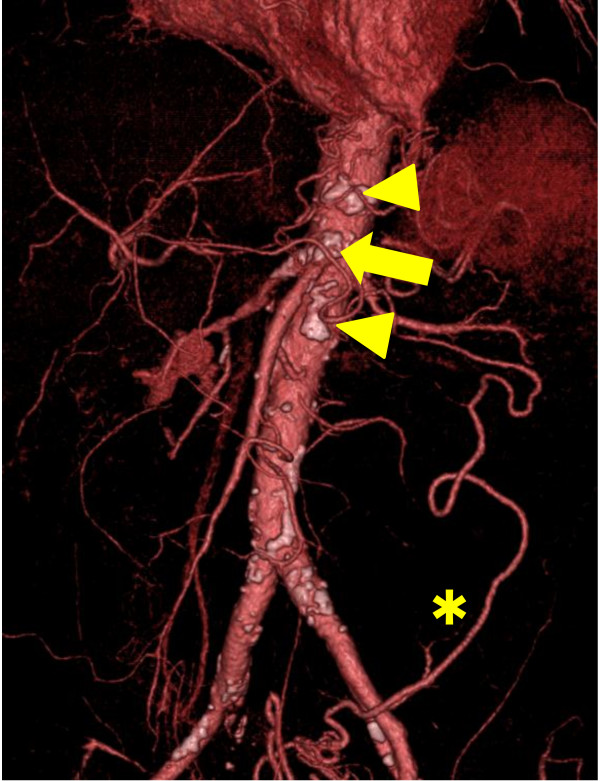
Computed tomography before the intervention. The arrow indicates occlusion of the superior mesenteric artery. The arrowheads indicate obstruction of the celiac and inferior mesenteric arteries. The asterisk shows the development of a collateral vessel from the left iliac artery.
